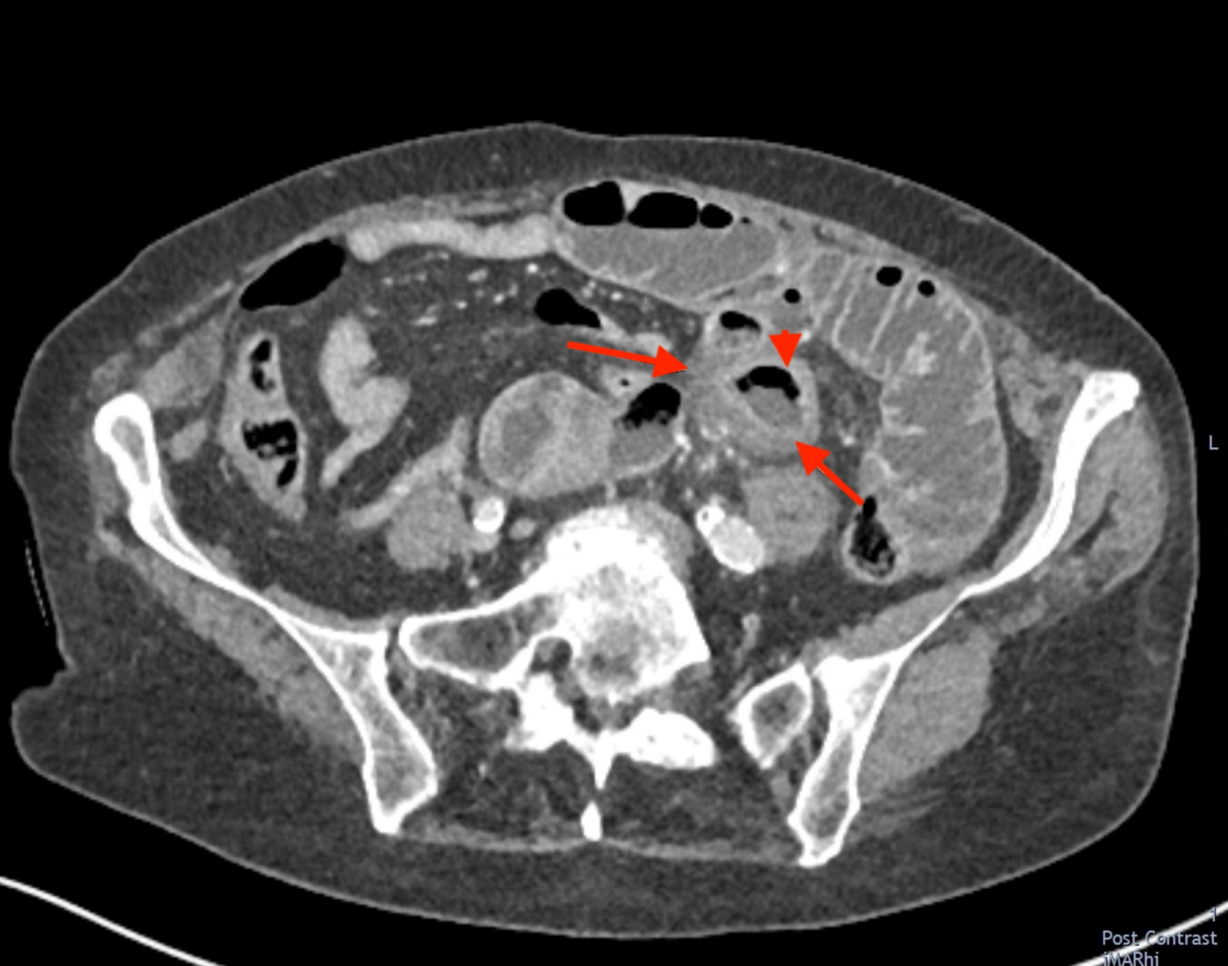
Axial CT image of an inflammed jejunal diverticulum (arrowhead). This is likely to be the originating site of the obstructing enterolith. The arrows highlight features of acute inflammation with wall thickening and surrounding mesenteric inflammatory stranding.
